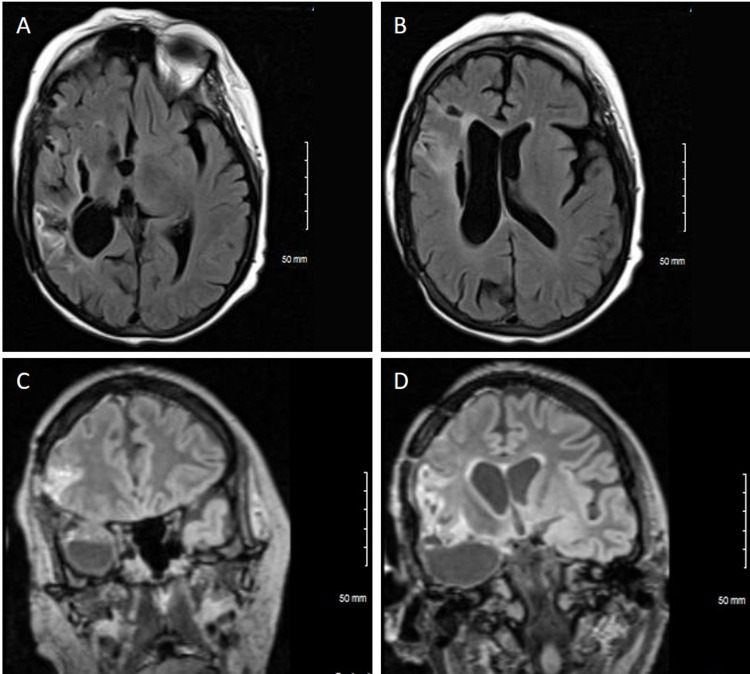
Brain MRI of the patient eight months after right middle cerebral artery territory stroke Axial (A, B) and coronal (C, D) T2/FLAIR MR sequences demonstrate encephalomalacia and surrounding gliosis in the right middle cerebral artery territory, including the right corona radiata. Also seen is right temporal laminar necrosis, ex vacuo dilatation of the right lateral ventricle, and interval development of a rightward 5 mm midline shift secondary to chronic right cerebral convexity volume loss. Images shown in two different planes each (A, inferior; B, superior; C, anterior; D, posterior).
